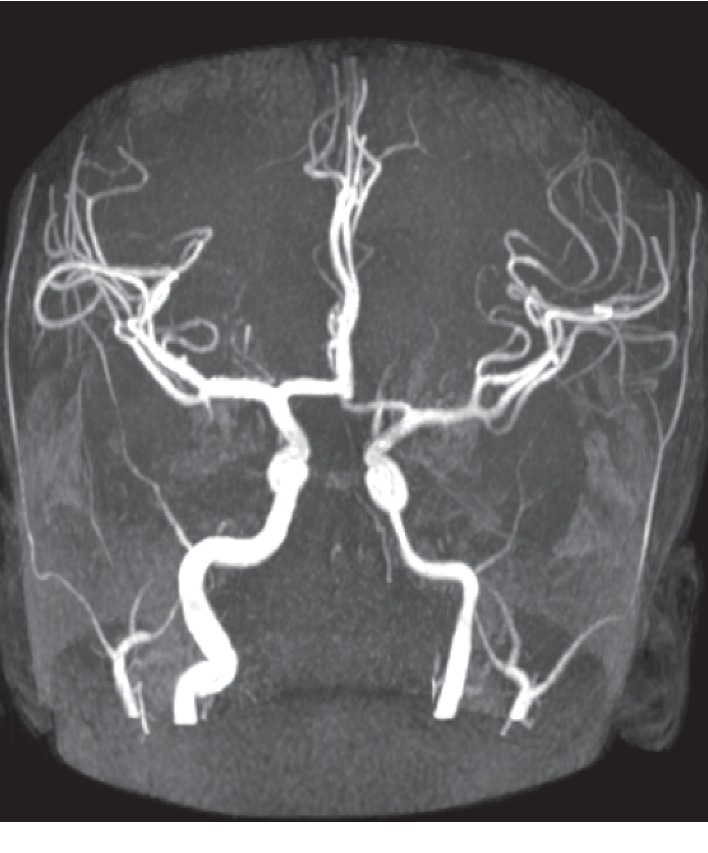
TOF 3D MRI angiography at admission, showing narrowing of the petrous, cavernous, and terminal segments of the left internal carotid artery corresponding to left internal carotid arteritis.
